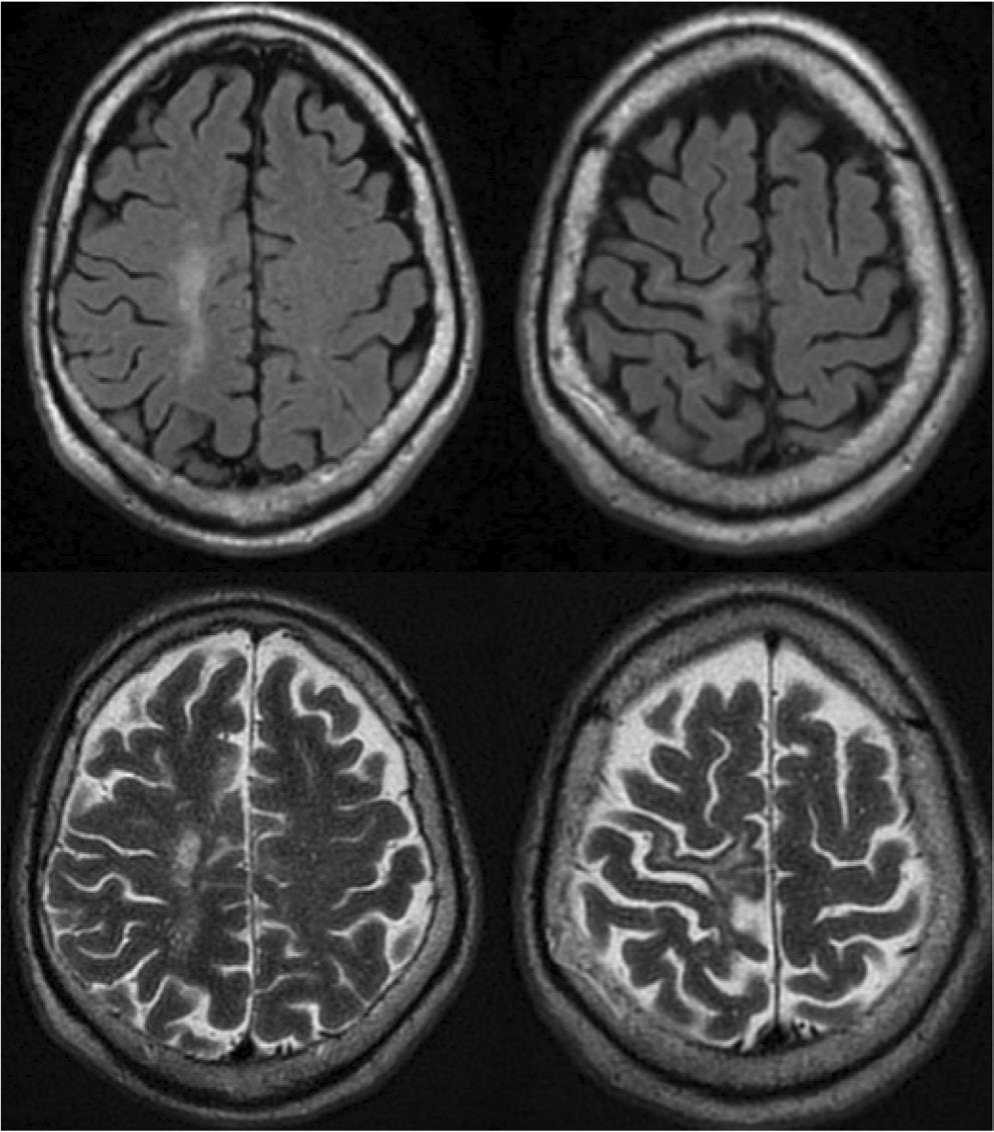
FIGURE 2 Axial FLAIR and T2‐weighted MRI after maraviroc treatment.
